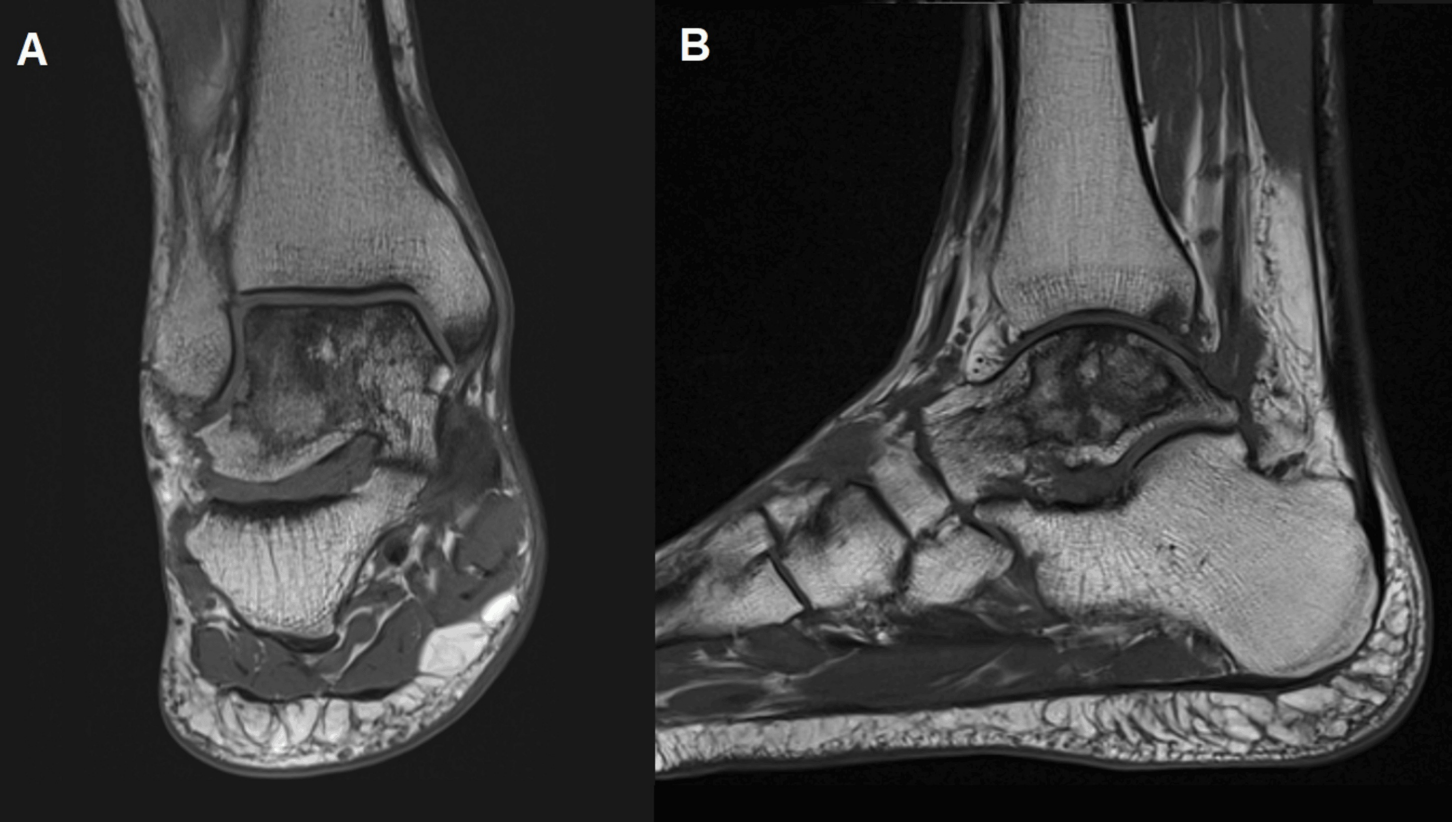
MRI T1-weighted images of the ankle in coronal (A) and sagittal view (B) at the time of the first evaluation. MRI exhibiting extensive bone infarctions of the talar head and body, occupying almost all of its volume. Focal chondropathy of the talus and distal tibial could also be identified.
